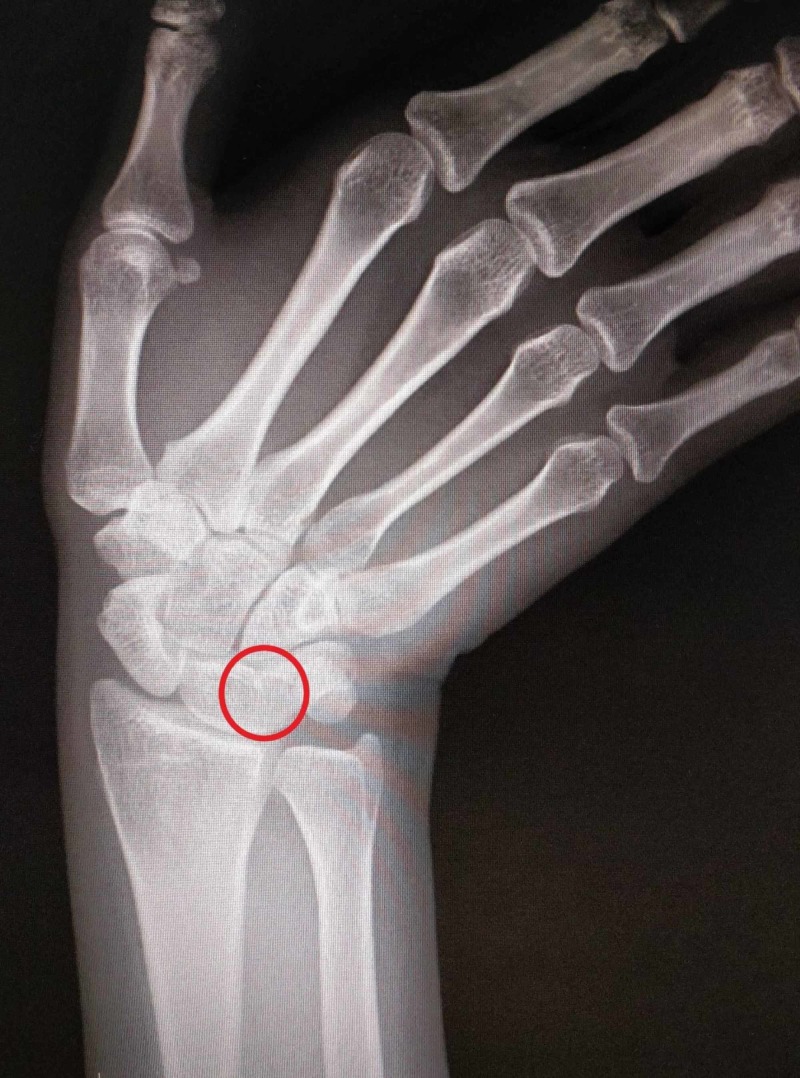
Lateral radiograph Coalition is marked in red circle
